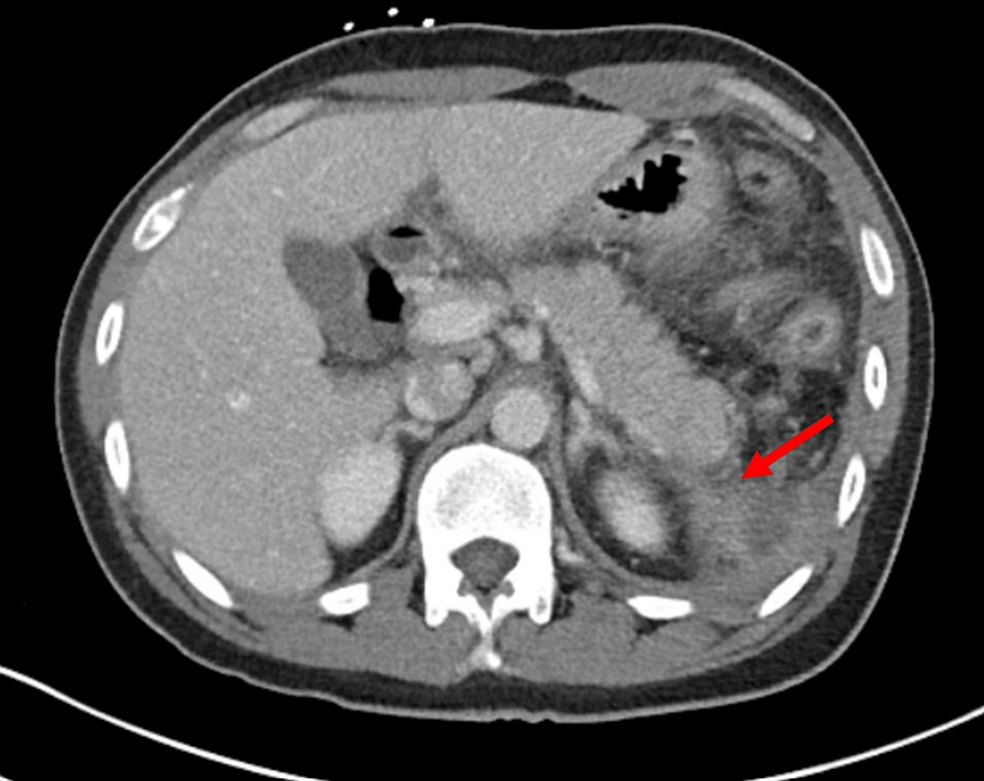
Computed tomography of the abdomen showing an accumulation of a subdiaphragmatic fluid collection (red arrow) on admission.
